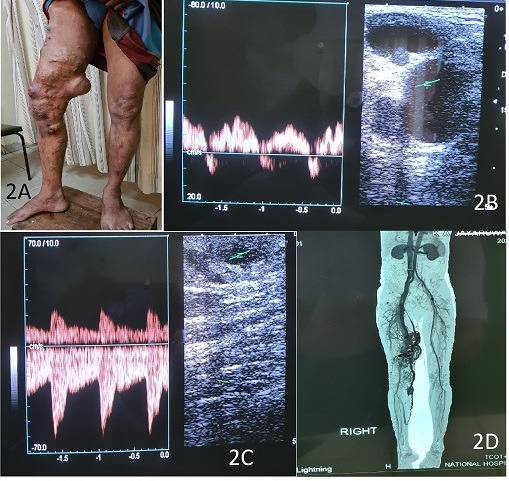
(A) Abnormal right lower limb VV; (B) arterial type flow at the SFJ; and (C) superficial veins; (D) CT angiogram depicting the AVM.
